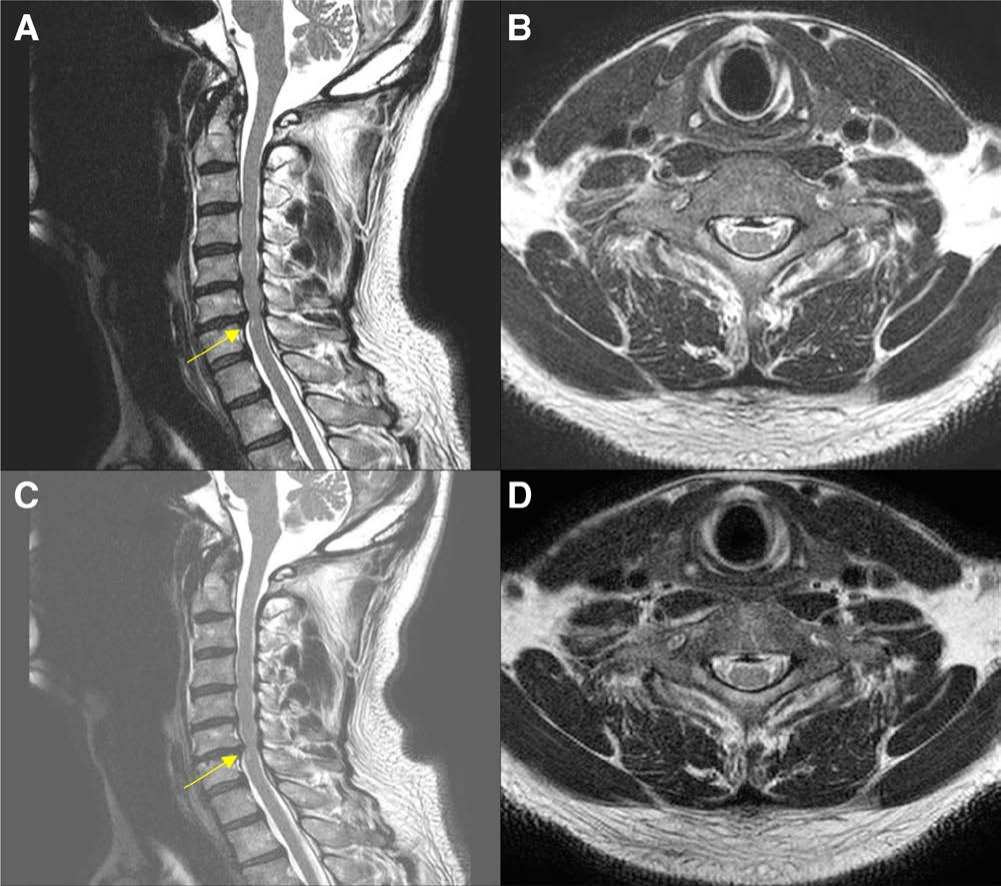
Case 1: (A) C-SPINE MRI. (B) Scanned on April 18, 2023. (C and D) Scanned on March 26, 2024. MRI = magnetic resonance imaging.
